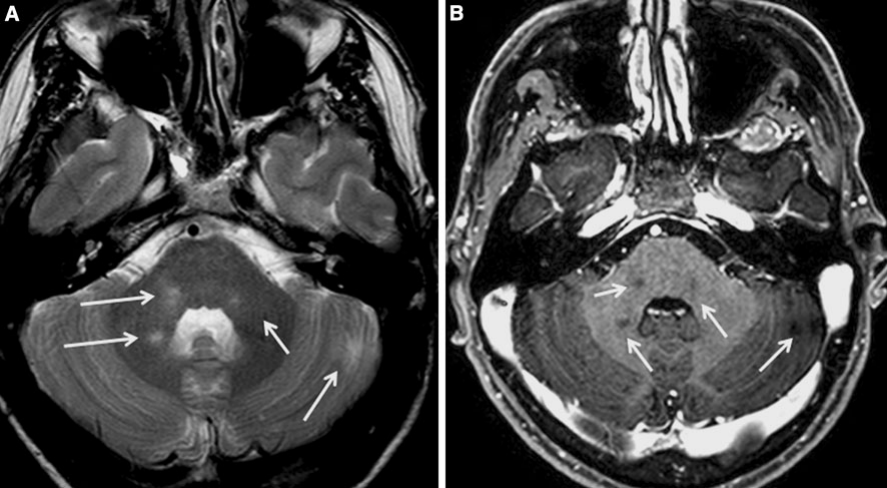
This MRI study was performed 2 years after that of Fig. 1 and showed new T2-weighted lesions, which are hypointense in T1 gadolinium-enhanced images in the pons and cerebellum (*arrows*). Hyperintense T2 lesions give a mirror image of the trigeminal root inlet and main sensory nucleus. a T2-weighted images; b T1 gadolinium-enhanced image
